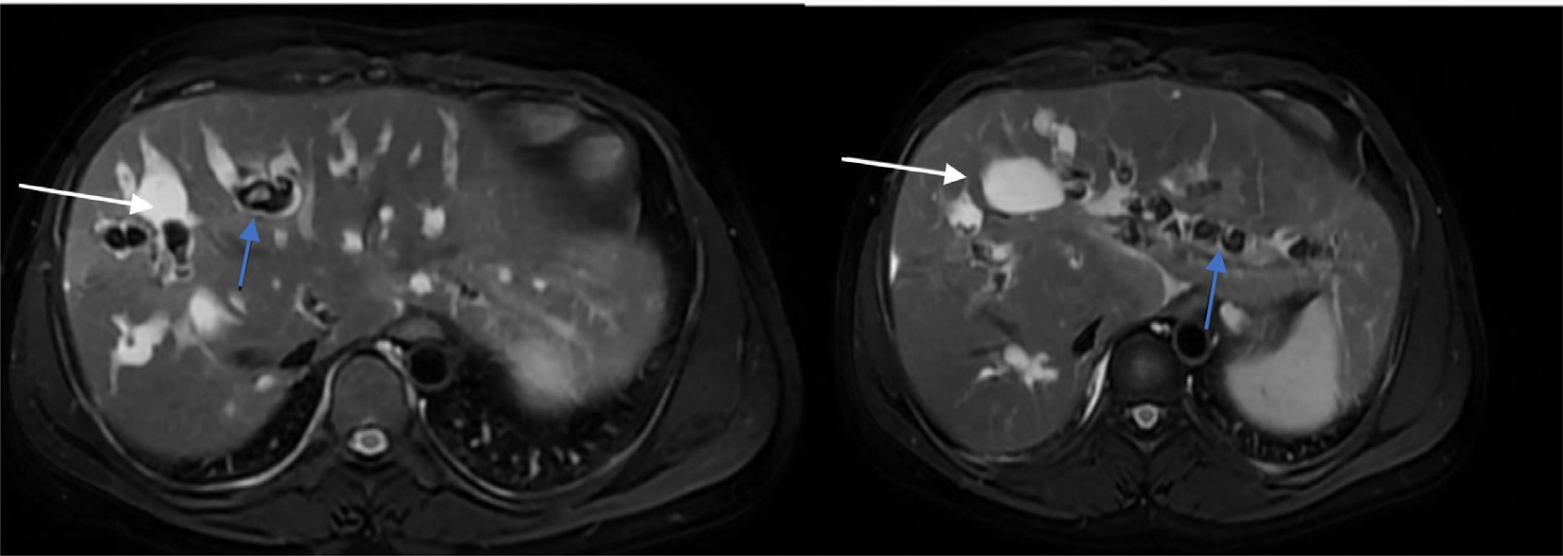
The axial T2-weighted MRI image shows multiple multifocal saccular cystic dilations of the intrahepatic bile ducts (white arrows) containing numerous stones (blue arrows).
